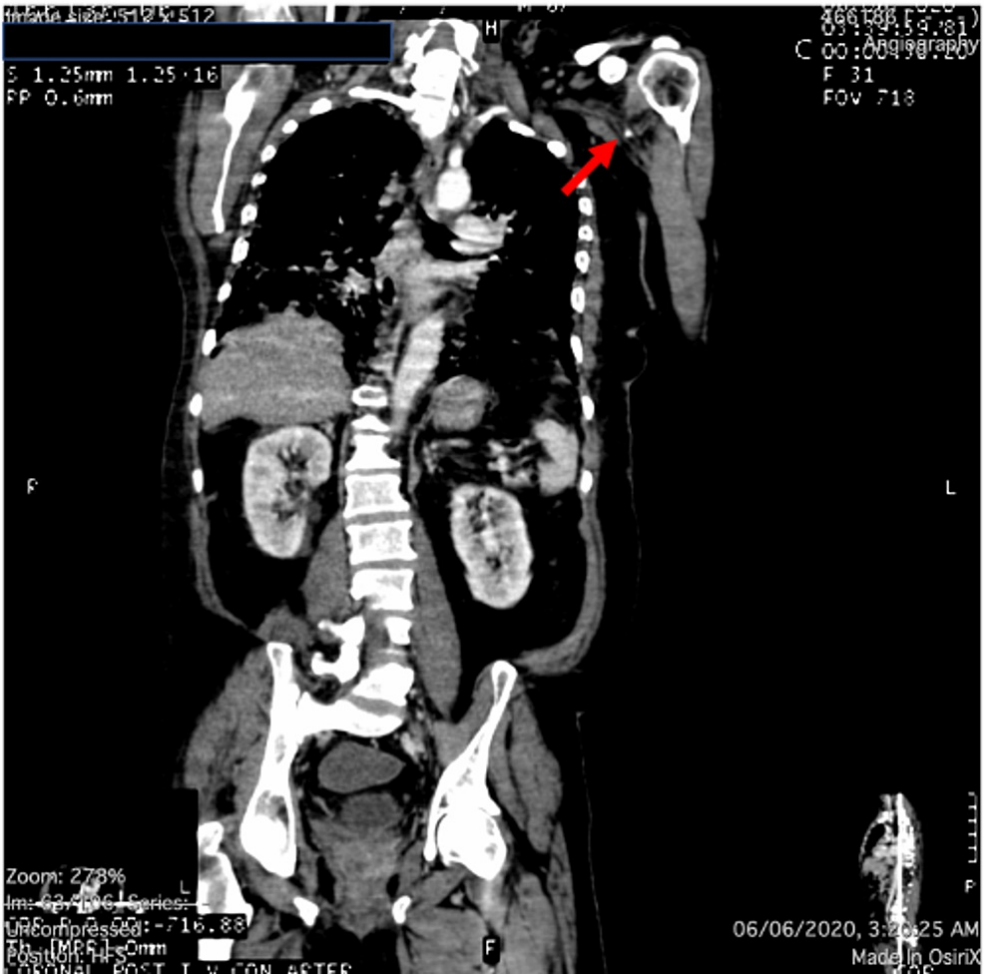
Coronal section of CT aortogram showing cut-off sign of the contrast at the level of junction between the left subclavian artery and the axillary artery (red arrow).
